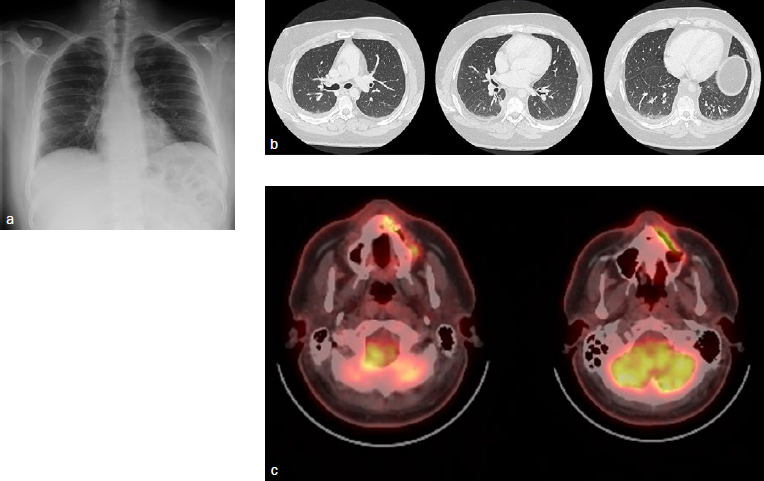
a) Thoracic radiograph: Discrete bilateral pleural effusion with subjacent parenchyma atelectasis. The remaining parenchyma has no significant alterations. b) Thoracic CT: Bilateral pleural effusion with areas of atelectasis in the subjacent parenchyma. Ganglia of unspecific size in the mediastinal territory with no signs of adenopathy. c) PET-CT of maxilla: hypermetabolism in left maxilla related with an increase of parts density and a fistulous tract in relation to an infectious-inflammatory process. Small submandibular laterocervical adenopathy (slightly contrast enhanced), related to a reactive-inflammatory process.
